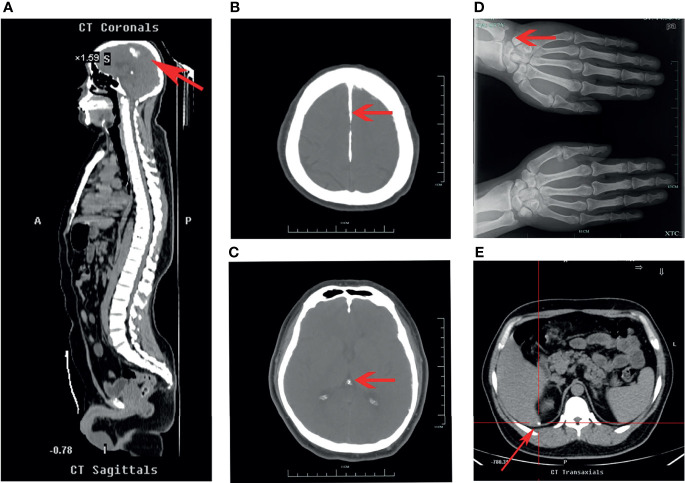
Upper half of body CT, head CT, and X-ray images of the both hands of the patient. (A, B) Upper half of body CT and head CT scans, respectively. Red arrows both indicate calcification of cerebral falx. (C) Head CT scan. Red arrow indicates calcification of tentorium cerebelli. (D) X-ray of both hands. Red arrow indicates calcium deposition. (E) Abdominal CT scan. Red arrow indicates calcification in the subcapsular region of the posterior segment of the right liver lobe.
